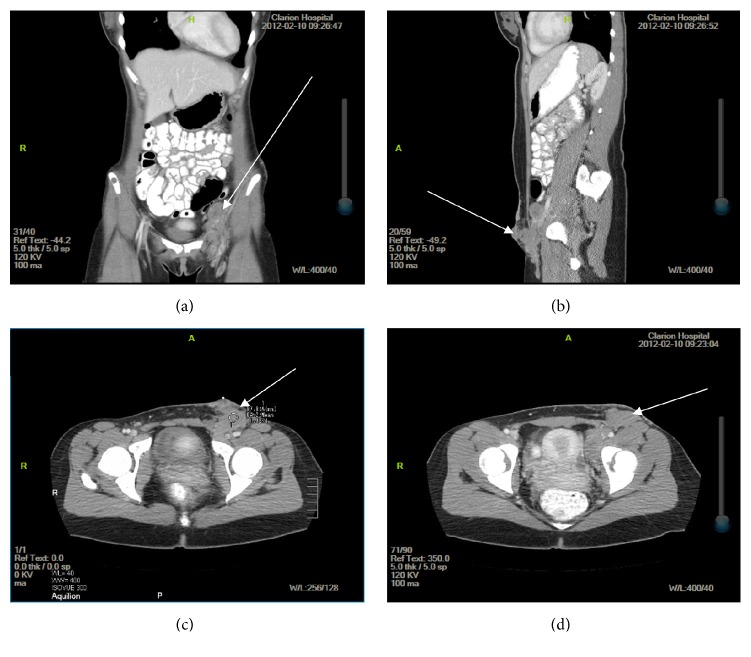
Initial CT imaging of the abdomen and pelvis with and without contrast for evaluation of the inguinal mass in (a) coronal, (b) sagittal, and (c) axial views demonstrating superficial extension and involvement of anterior abdominal wall by mass and (d) axial view demonstrating a proximal cross-sectional view of the mass that is not extending to the superficial skin.
